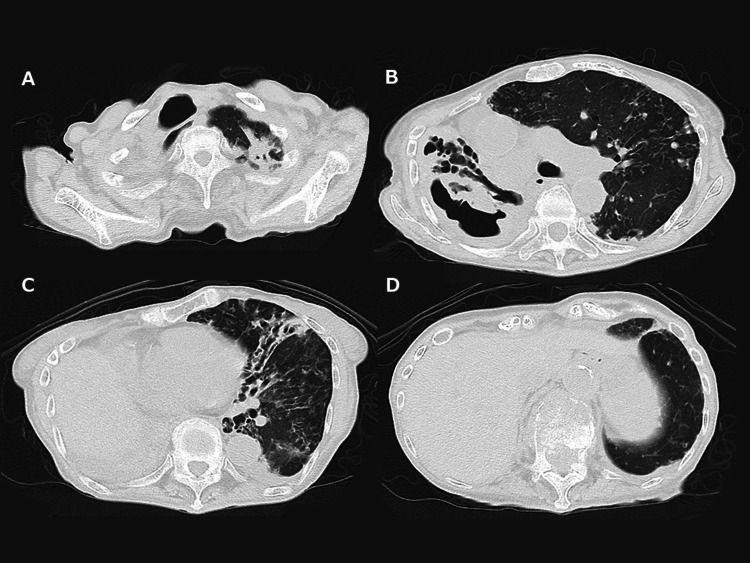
Chest computed tomography scan on admission. A-D: The right lung shows findings compatible with chronic necrotizing pulmonary aspergillosis, such as bronchiectasis, cavities, and infiltrative shadows. The left lung shows findings compatible with pulmonary *Mycobacterium avium* complex disease, such as multiple bronchiectasis, granular shadows, nodular shadows, and cavities.
